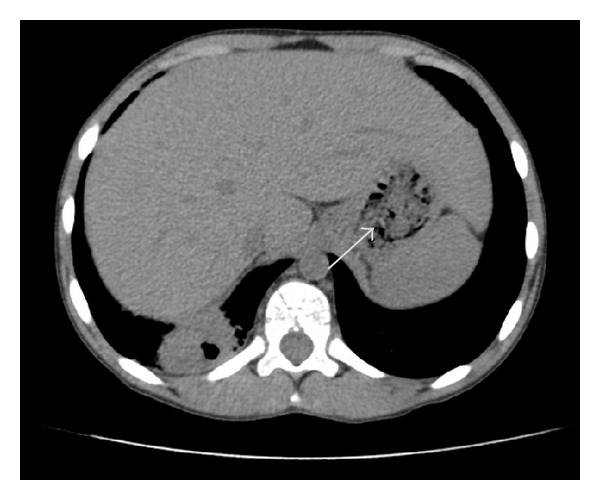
Chest computed tomography showing cavitation's of the left lung.
